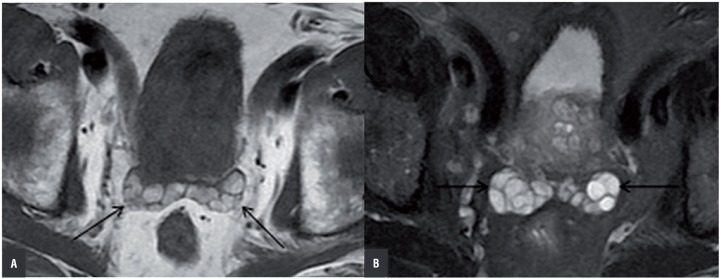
Bilateral SV hemorrhage in a 75 year old patient with BPH. Axial (a) T1 weighted MR image, axial (b) fat-saturated T2 weighted MR image show; hyperintense signal features on both SVs in T1 and T2 weighted series (black arrows).
